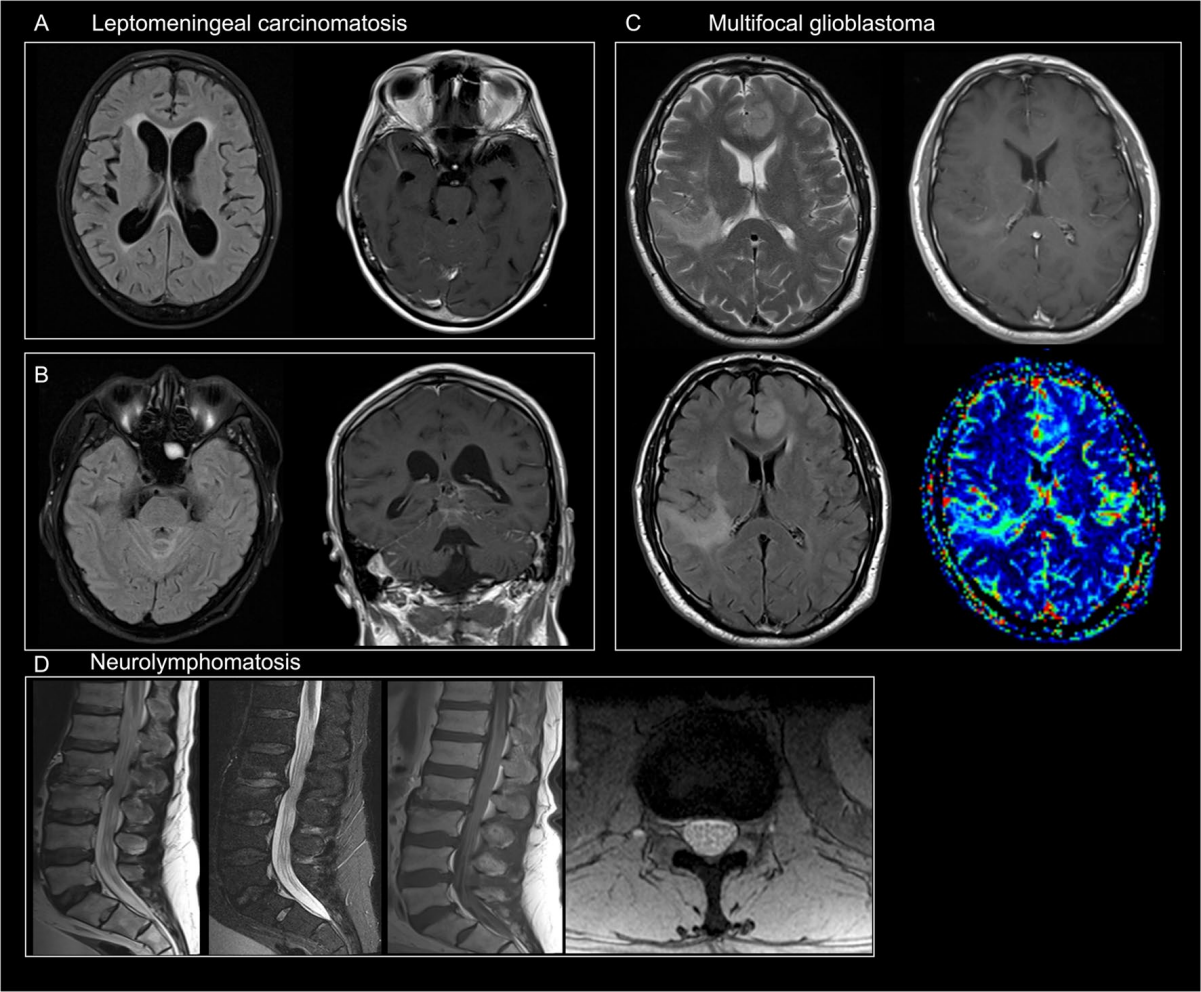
Imaging examples of patients who were initially thought to have PNS but later had an alternative neoplastic diagnosis. A and B Two distinct cases of leptomeningeal carcinomatosis are shown. In case A, axial FLAIR images demonstrated moderate dilation of the lateral ventricles, associated with periventricular hyperintensities, particularly at the level of the frontal horns. Post-gadolinium SE T1-weighted images revealed enhancement along the vermis and cerebellar sulci. In case B, a bright signal along the cerebellar sulci was seen on axial FLAIR images, while diffuse leptomeningeal enhancement was observed on coronal post-gadolinium SE T1-weighted images. C Multiple hyperintense lesions on T2-weighted and FLAIR images involved both the cortex and white matter of the left frontal lobe and right temporal lobe. No contrast enhancement was seen on post-gadolinium SE T1-weighted images; however, perfusion evaluation revealed a significant increase in relative cerebral blood volume (rCBV) in the same areas. The patient was diagnosed with a biopsy-proven multifocal glioblastoma. D Sagittal TSE, Short TI Inversion Recovery (STIR) T2-weighted, post-gadolinium TSE T1-weighted, and axial Gradient Echo (GE) T2-weighted images showed diffuse thickening of the cauda equina nerve roots. Mild enhancement was noted on T1-weighted images. The spinal canal diameter was normal, and no focal disk herniations were detected. The patient had non-Hodgkin lymphoma (NHL) and was diagnosed with neurolymphomatosis
